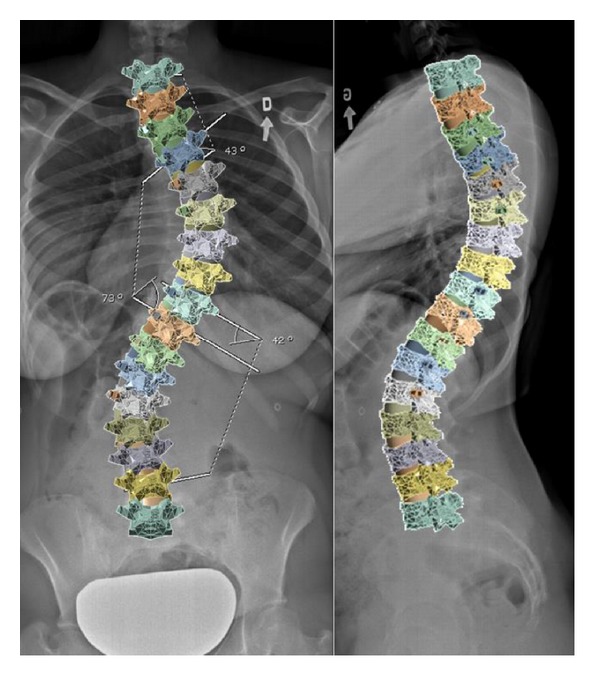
Coronal and sagittal radiographs (thoracic curve 73°, kyphosis 35°, and lordosis 37°) and superimposed FEM (thoracic curve 69°, kyphosis 32°, and lordosis 36°).
